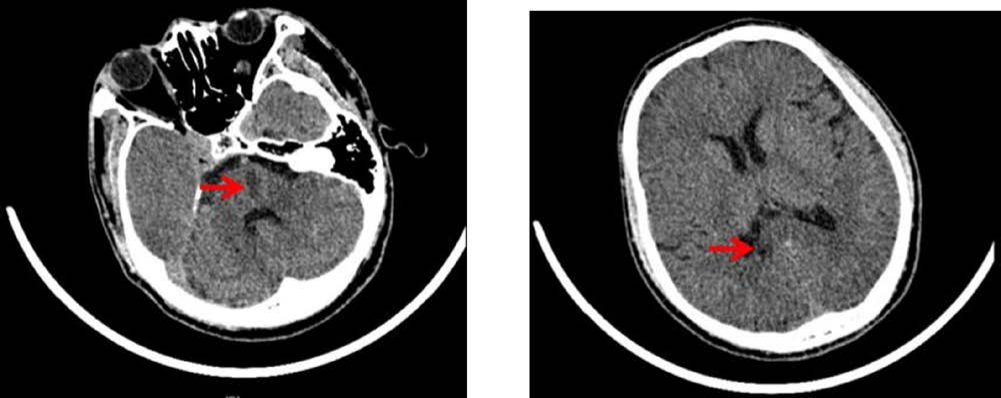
2021-11-03 Computed tomography of the head revealed suspicious hypodense shadows in the pons and corpus callosum.
